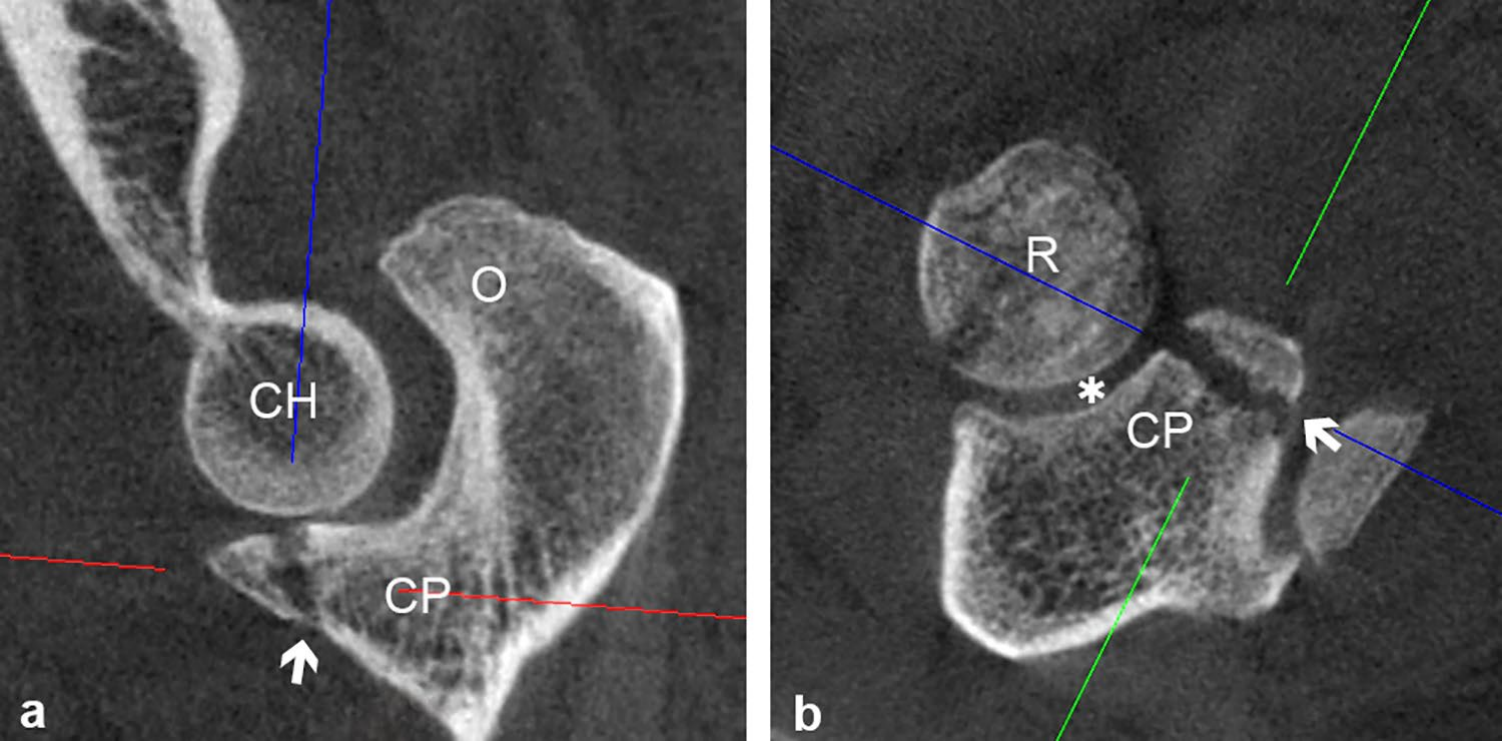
a,  b CT scan (a: sagittal view; b: axial view) of an elbow joint of a 52-year-old male with an anterolateral fracture of the coronoid process and fracture of the radial head. The white arrows denote the fracture line. The fracture line corresponds to approximately 50% of the height of the coronoid process (Regan and Morrey Type 2 fracture) and also involves the proximal radioulnar joint (white star). *CP* coronoid process, *R* radial head, *CH* capitulum humeri, *O* olecranon
